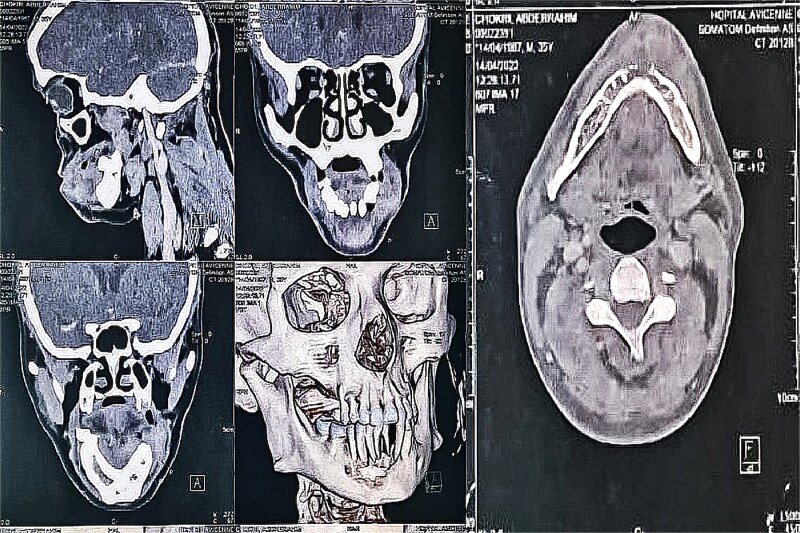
CT scan of the facial mass with axial, coronal, and sagittal slices, as well as 3D reconstructions, showing the tumor and its aggressiveness with cortical lysis at the symphysis.
